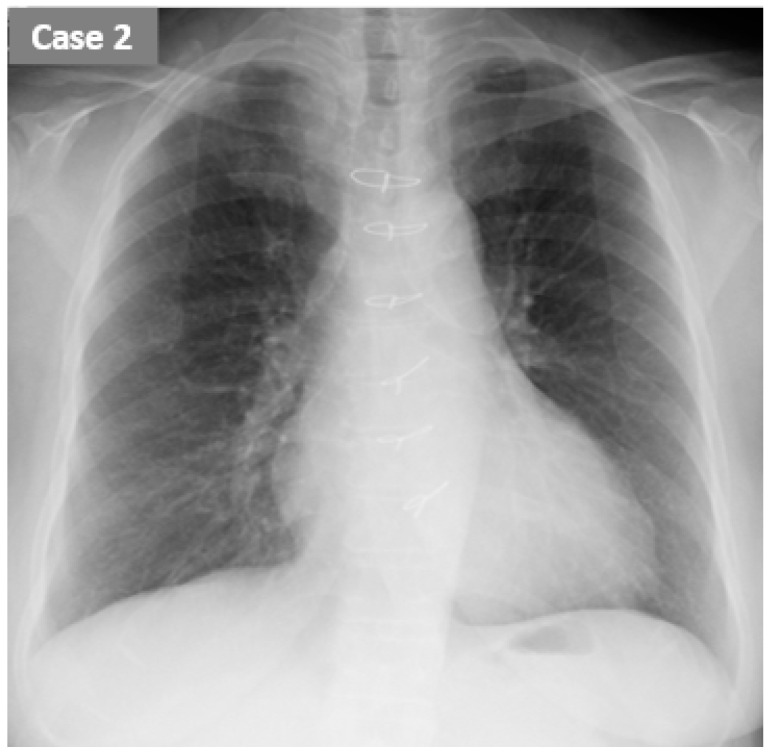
The chest X-ray just before hemodialysis in case 2.
